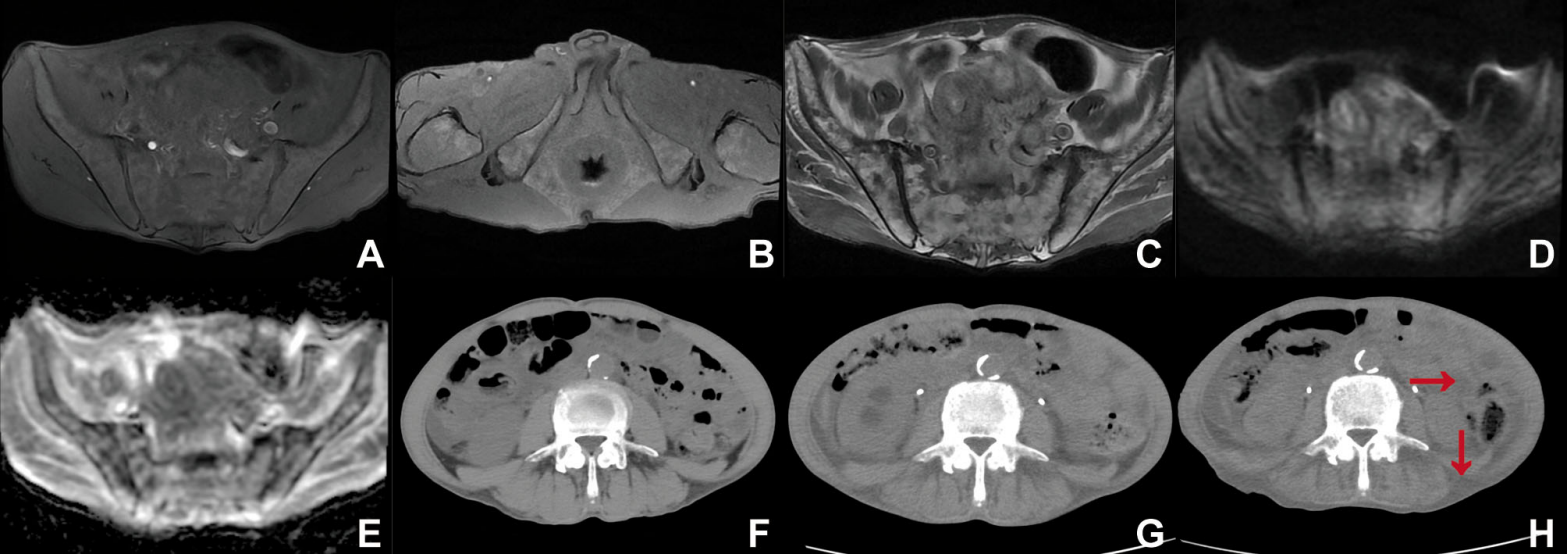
(A) T1WI (sacroiliac joint level), (B) T1WI (ischiorectal fossa level), (C) Fat-suppressed T2WI (T2WI-SPAIR), (D) DWI (b=800 s/mm^2^), (E) ADC map, (F–H) Abdominal computed tomography (CT) scans were conducted seven months ago (F), three months ago (G), and at present (H), utilizing a window width (WW) of 350 and a window level (WL) of 40.
